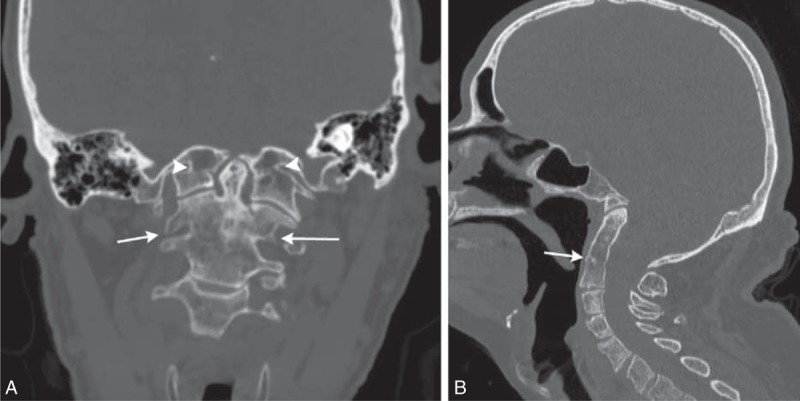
(A) Coronal reformat of CT examination. Platybasia–odontoid process is above bimastoid line. Also note atlantooccipital assimilation (arrowheads) and assimilation of C2 and C3 (arrows). (B) Sagittal reformat of CT examination. Platybasia—angle of skull base is 155 degrees, odontoid process is 25 mm above Chamberlain line. Assimilation of C2 and C3 (arrow). CT = computed tomography.
